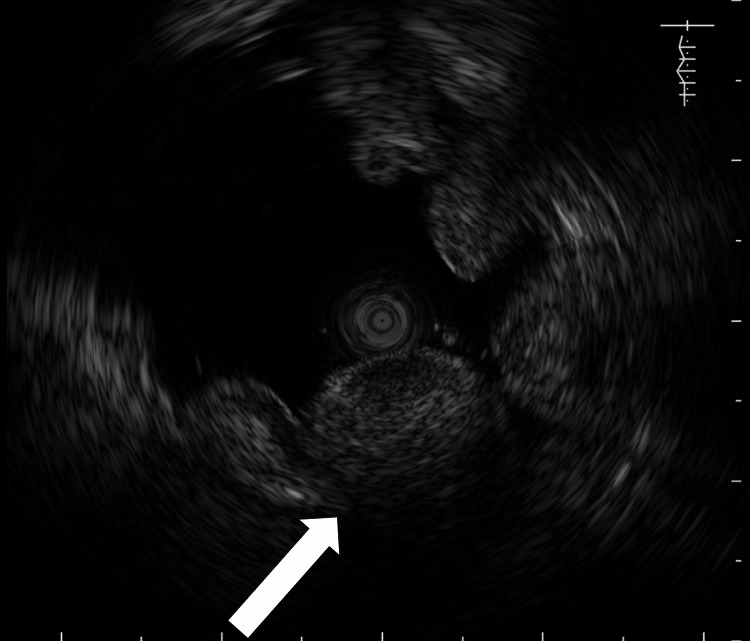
Preoperative ultrasound endoscopy Preoperative ultrasound endoscopy is diagnostic of advanced cancer of gastric submucosal cancer.
